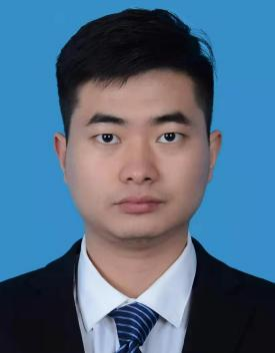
Lei Liu is an attending physician in the Department of Ultrasound Medicine at Nanjing Gulou Hospital. He has 8 years of clinical experience and has been practicing cardiac ultrasound diagnosis for more than 6 years. He has published several papers on cardiac Science Citation Index.
